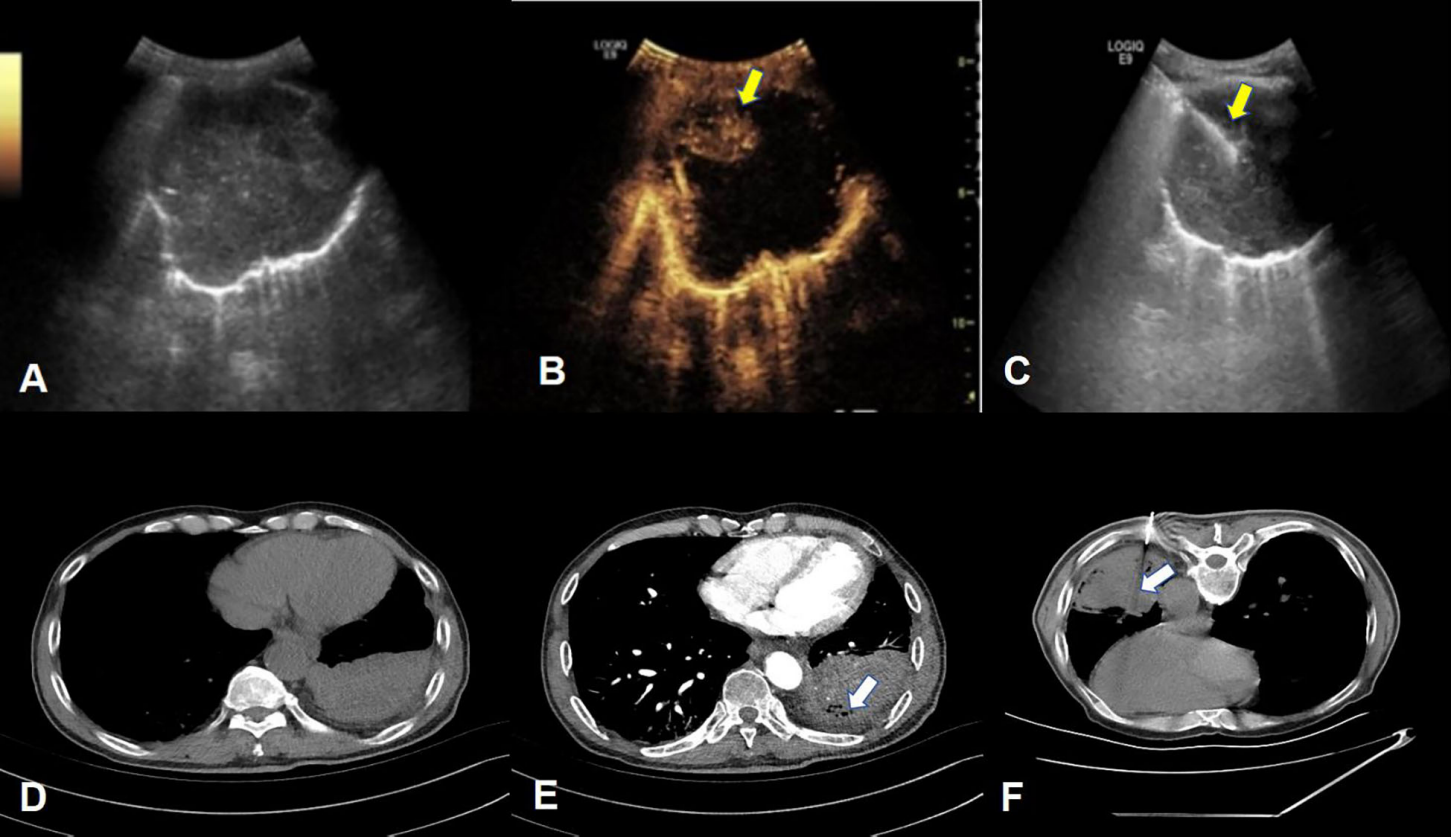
Example of percutaneous lung biopsy guided by contrast-enhanced ultrasound and contrast-enhanced CT. (A–C) respectively show the two-dimensional gray-scale ultrasound, contrast-enhanced ultrasound and ultrasound-guided puncture needle tract of PPLs. (B) clearly shows extensive liquefaction necrosis in the lesion without enhancement. The puncture needle tract shown in (C) avoids the non-enhancement area. (yellow arrow), the final pathological result of this patient was lung squamous cell carcinoma; (D–F), respectively show the normal CT, enhanced CT and CT-guided puncture needle path of the lesion, (E) shows the local non-enhancing area within the lesion (white arrow), Figure The puncture needle track shown in (F) passed through the non-enhancing area and reached the deep tumor-enhancing area. The final pathological result of this patient was lung adenocarcinoma.
